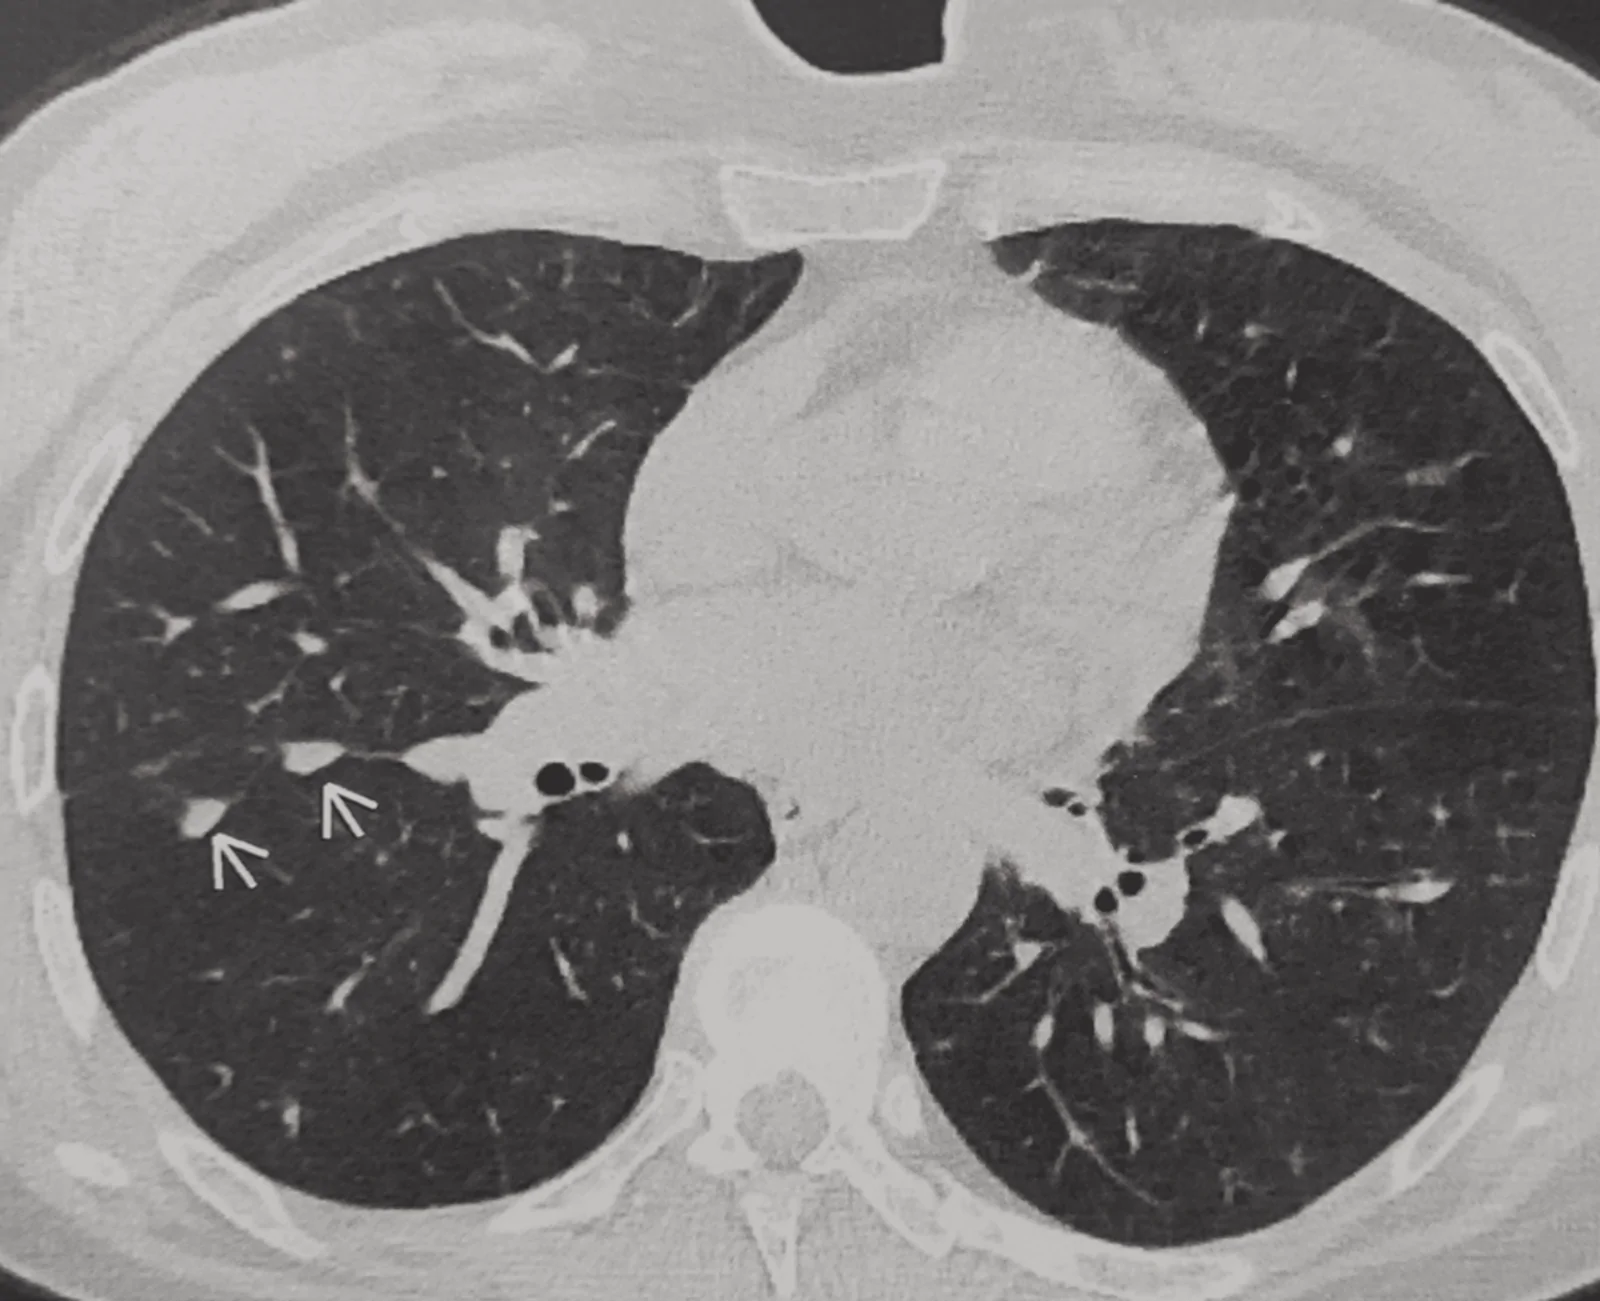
Follow-up chest computed tomography (CT) scan (2025, lung window) Complete resolution of the tree-in-bud micronodules following antibiotic therapy, with the appearance of two small nodules in the right upper lobe
